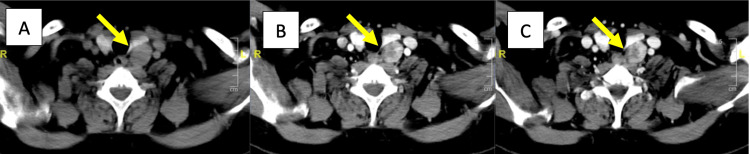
4D CT Scan for Parathyroid Lesion in a 46-Year-Old Man. 4D CT scan depicting a left inferior parathyroid adenoma measuring 3.6×2.1×2.1 cm, visualized in the plain (A), arterial (B), and venous (C) phases. The lesion demonstrates attenuation values of 48 HU, 112 HU, and 72 HU, respectively, across these phases. The percentage arterial enhancement (PAE) of the lesion was calculated to be 147.6%. 4D CT, four-dimensional computed tomography; HU, Hounsfield units
